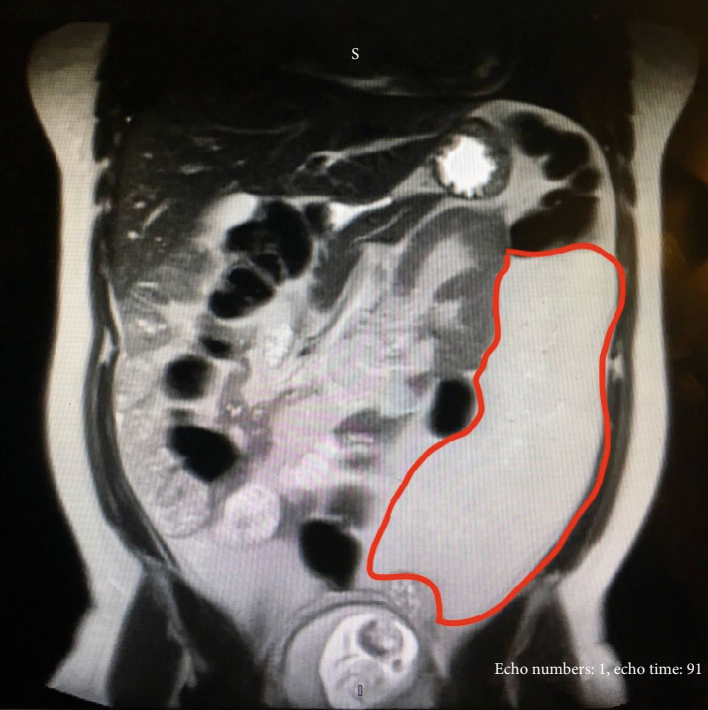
T2-weighted MRI at 15-week gestation demonstrates large homogeneous fat-containing mass which measured 28 × 14 × 6 cm with mass effects of the bowel. A minimal amount of intralesional fluid is noted; no irregular septa are identified, consistent with a simple lipoma.
